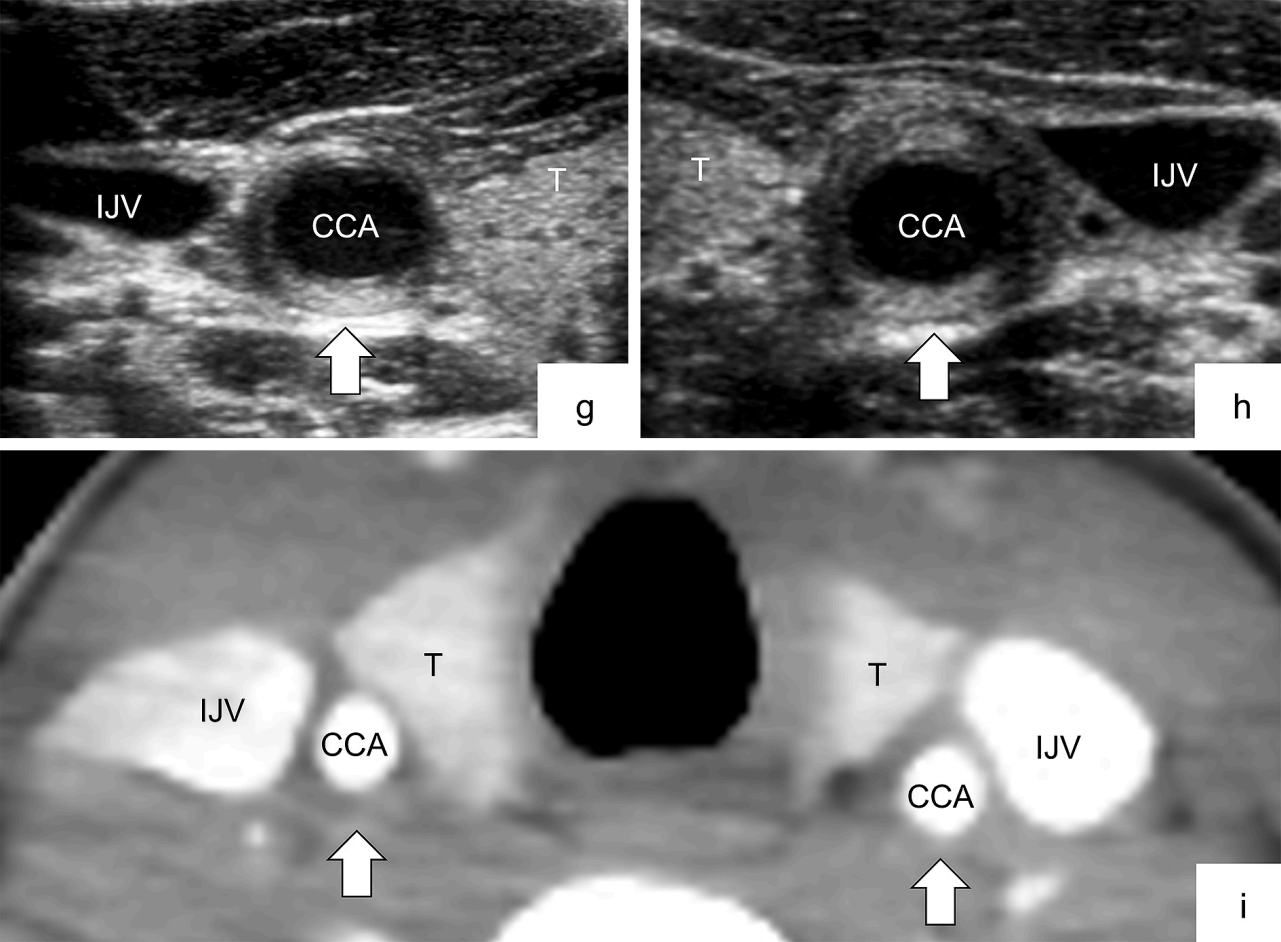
Transverse imaging of bilateral CCAs in Patient No. 4 (panels g to i). g and h: Thickening at the dorsal walls of bilateral CCAs is clearly detected via ultrasonography (panel g shows the right side, and panel h shows the left side). i: Wall thickening at the same level is unclear in CECT. CCA, common carotid artery; IJV, internal jugular vein; T, thyroid gland.
